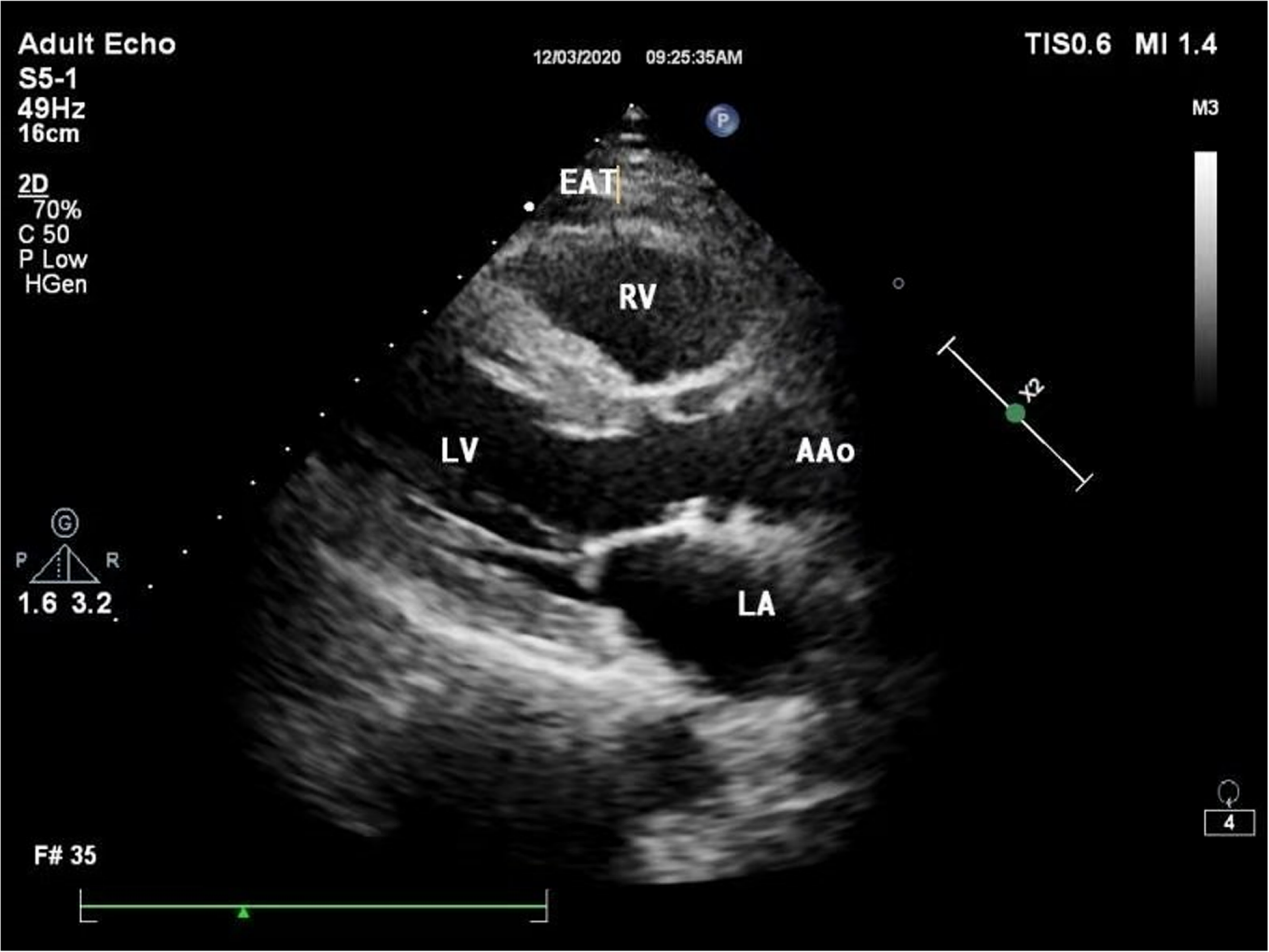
The thickness of EAT was measured by ultrasound. In the parasternal long-axis view, the EAT thickness of the free wall of the right ventricle was measured at the end of diastole. EAT: epicardial adipose tissue; RV: right ventricular; LV: left ventricular; AAo: ascending aorta; LA: left atrium
